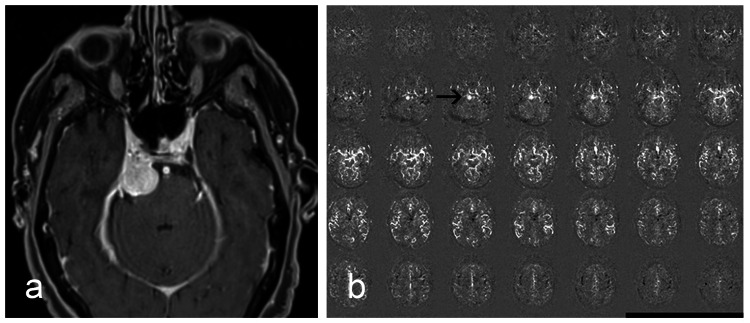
Meningioma in the right cerebellopontine angle. (a) Contrast-enhanced T1-weighted image. (b) ASL mosaic images with increased signal in the area of the tumour (black arrow). Meningioma is clearly visible despite the global ASL perfusion signal decline in the brain.
